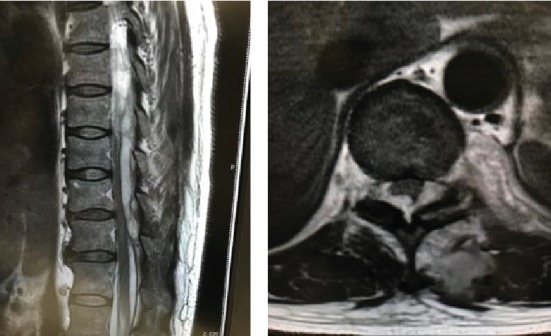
T2-weighted sagittal and axial view showed a facet effusion, paraspinal abscess, and epidural abscess in the level of 10th-11th thoracic vertebra.
